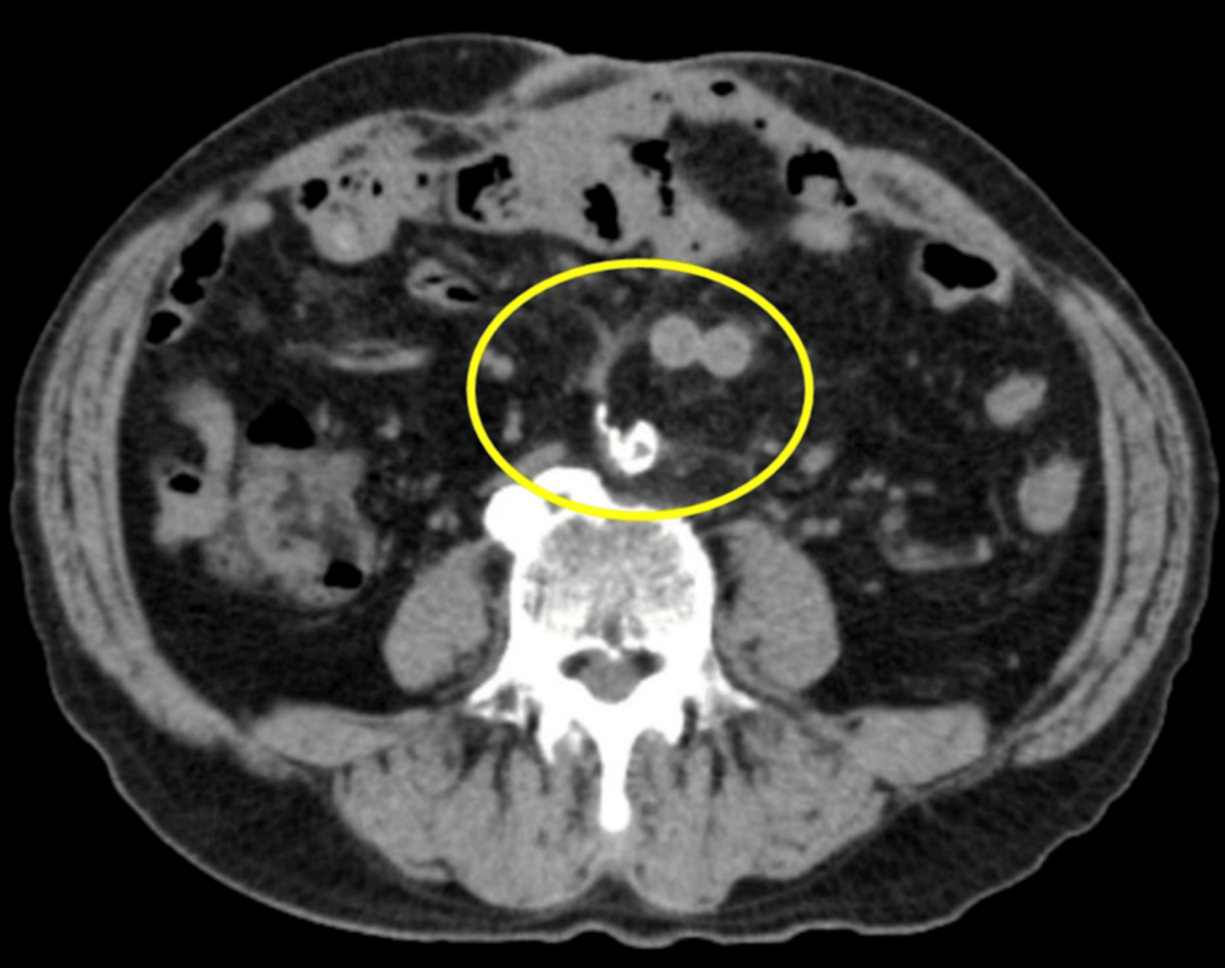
Plain CT of the abdomen and pelvis at 16 weeks The scan shows progressive resolution of fluid accumulation around the prosthetic graft (yellow circle).
